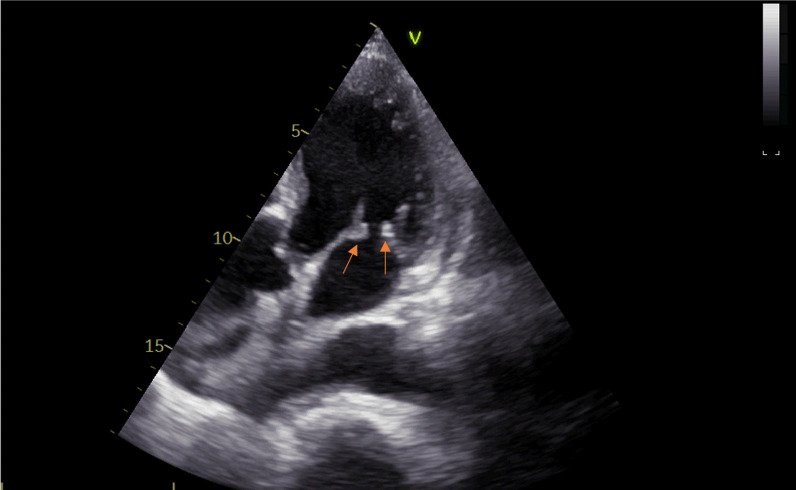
Echocardiographic image showing the 2 vegetations on the 2 mitral valves
